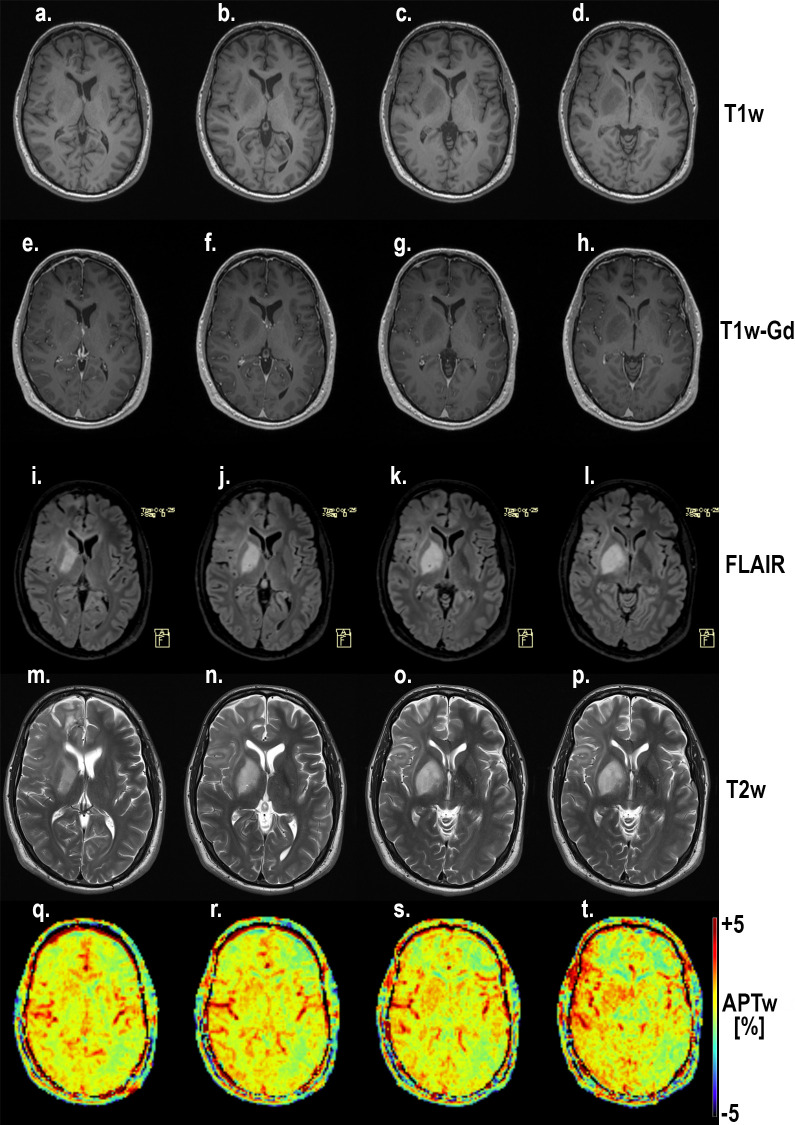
Four slices through the lesion of subject 5 with a high grade glioma (HGG). Notice the coherence between the increasing hypointensity on T1-MPRAGE (a-d), hyperintensity on FLAIR (e-h), increased hyperintensity on T2w MRI (i-l) and increased APTw signal (m-p). Also notice the increased APTw signal in blood vessel regions. Gd-enhancement was studied with T1w; T1-MPRAGE. Hyperintensities on T2 FLAIR and T2 turbo spin-echo of conventional pre-contrast protocols were correlated to T1w-Gd enhancement where possible, otherwise hypointensity.
